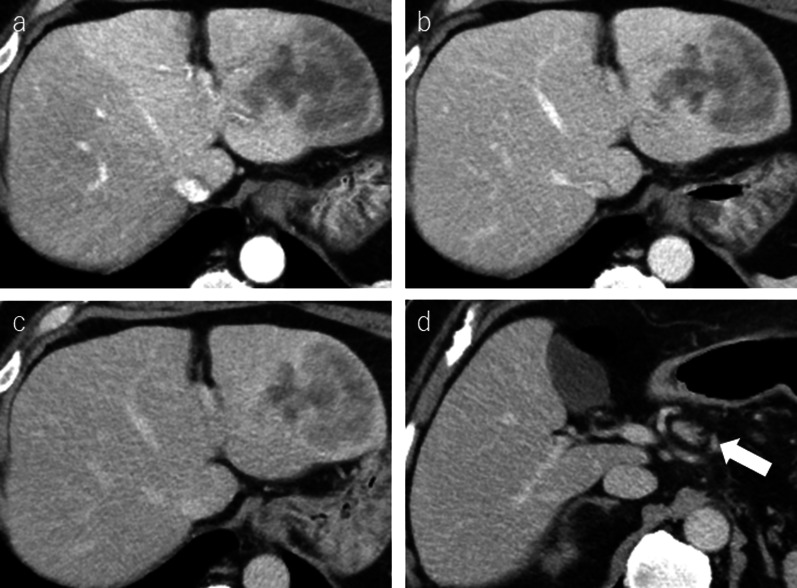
a–c Contrast-enhanced computed tomography shows an 80 mm heterogeneous hypovascular mass in the lateral section. The mass is gradually enhanced from early to late phase. d Lymph node of anterosuperior common hepatic artery is enlarged, suspicious of metastasis (arrow)
